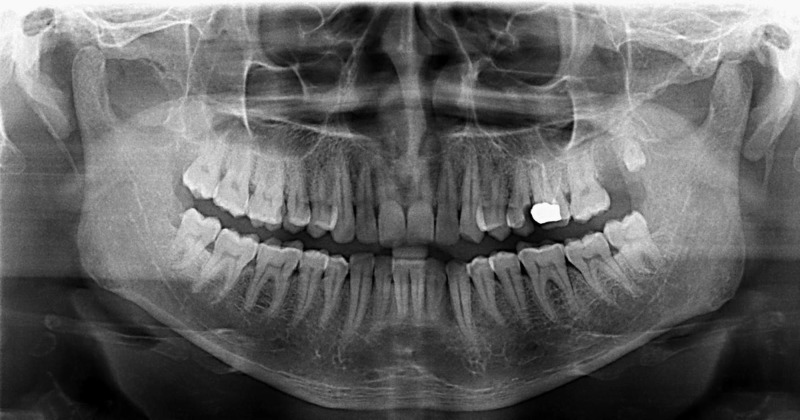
Orthopantomogram No evidence of bony erosions or gross pathology.
